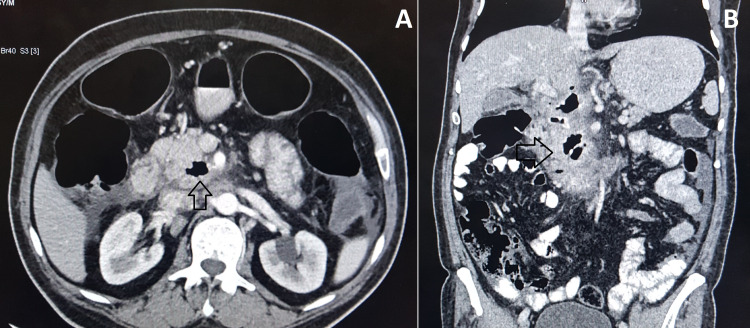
Contrast-enhanced computed tomography of the abdomen showing the hypodense lesion containing air foci (arrows) in the pancreatic head region with surrounding fat stranding in the axial (A) and coronal (B) sections.
